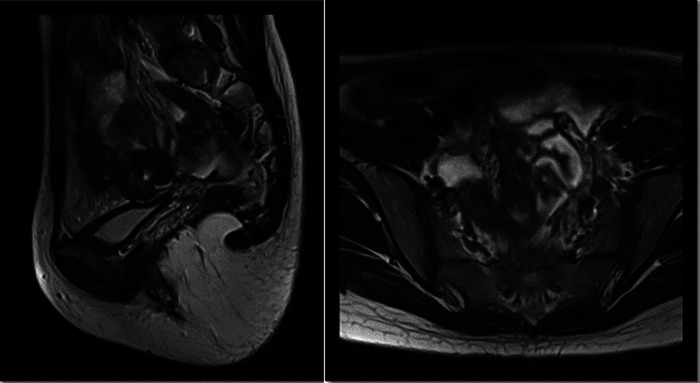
Postoperative pelvic magnetic resonance images (1 month after surgery).
